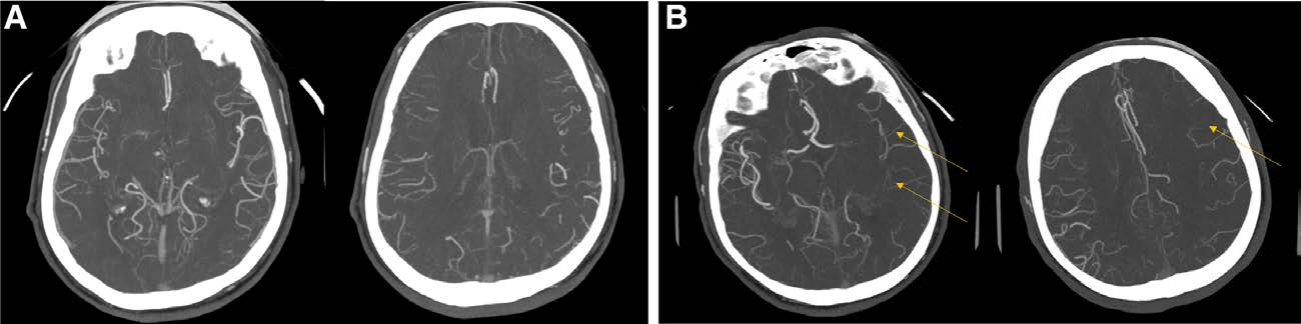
According to the study of Menon et al, examples of evaluation of collateral flow with computed tomography angiography (CTA). (A) a patient who was diagnosed with right M1 occlusion and underwent IAT, which was evaluated at 15 points and judged to be a good collateral channel. (B) a patient diagnosed with left distal ICA occlusion, which was evaluated as 9 points and judged to have a poor collateral channel. IAT = intra-arterial thrombectomy, ICA = internal carotid artery.
